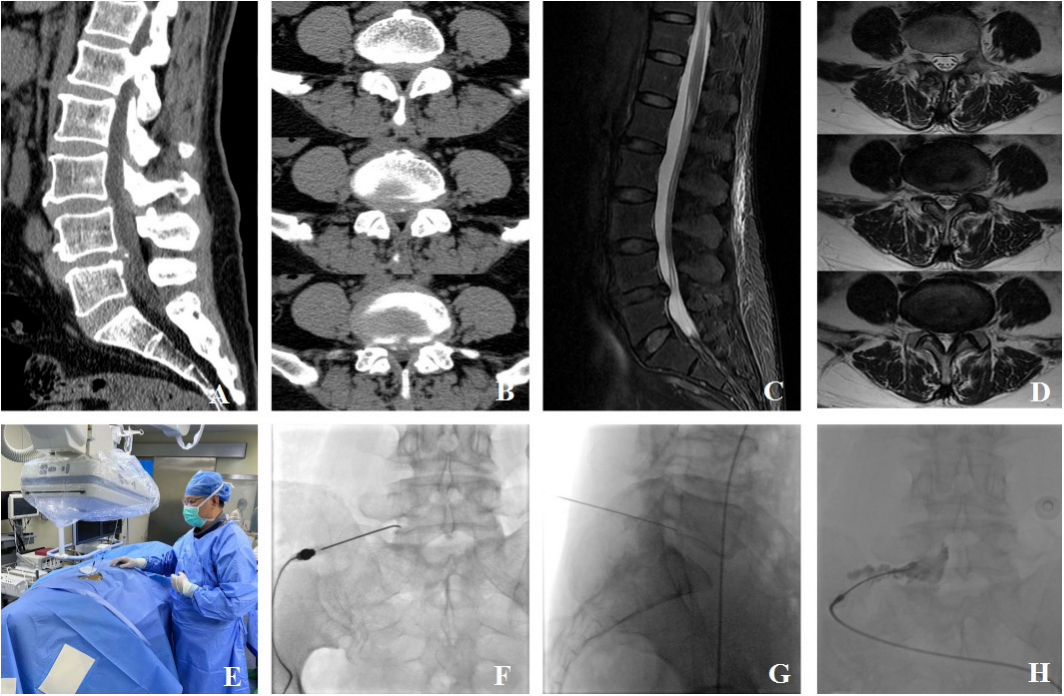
A typical case of DSA-guided radiofrequency pulsing combined with selective nerve root block. The patient is a 59-year-old female who was admitted due to “lumbosacral pain with numbness in the right lower limb for 4 months.” Lumbar spine CT scans in sagittal (A) and axial (B) planes revealed an L4/5 disc herniation (central-paracentral type) with calcification, right lateral recess stenosis, and posterior dural sac compression. Lumbar spine MRI scans in sagittal (C) and axial (D) planes also demonstrated an L4/5 disc herniation (central-paracentral type) with local subcutaneous soft tissue edema. (E) Intraoperative image of the DSA-guided radiofrequency pulsing procedure. (F,G) The radiofrequency needle puncturing under DSA guidance to reach the right L5/S1 intervertebral foramen. (H) A post-radiofrequency pulsing treatment image with iohexol contrast, showing the contrast agent traveling along the spinal nerve and within the vertebral canal.
